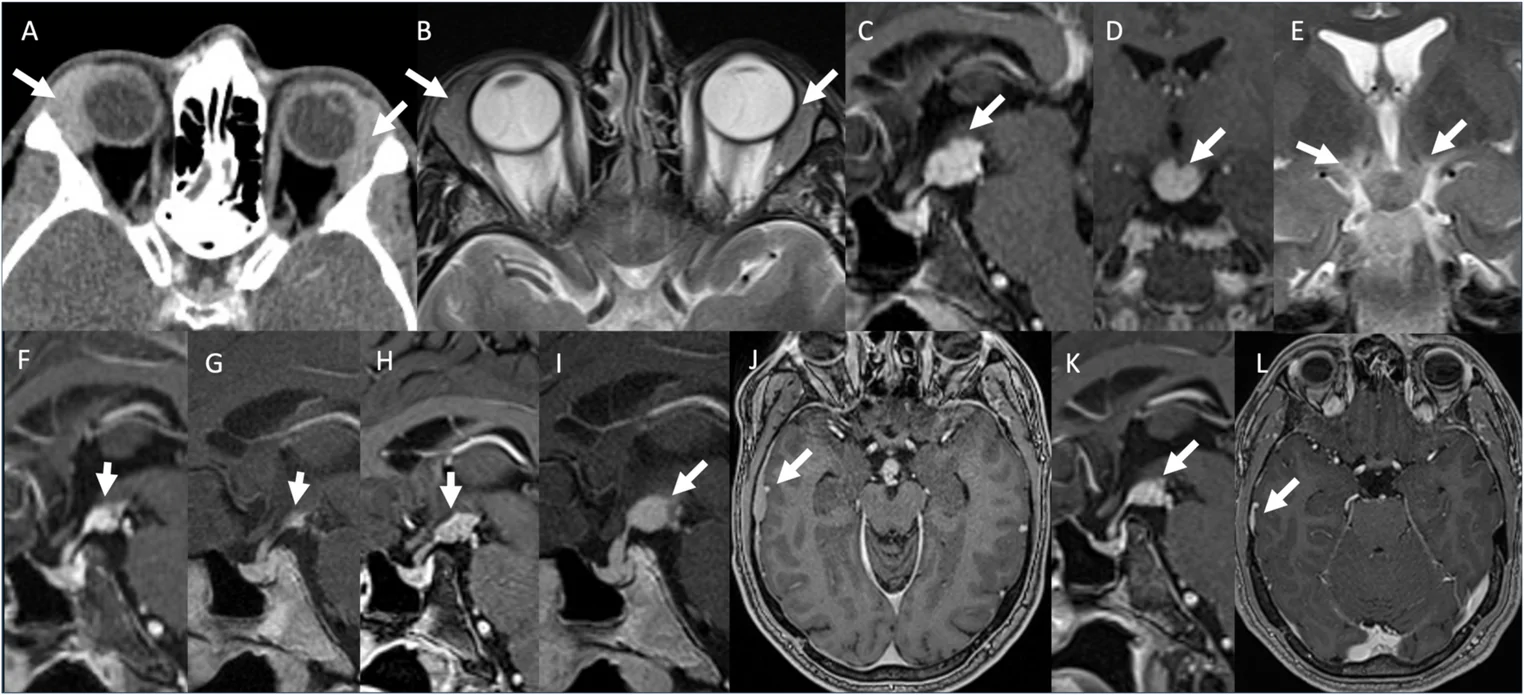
Radiological evolution of hypothalamic and dural involvement in a patient with IgG4-RD. A 33-year-old man with IgG4-RD initially presented with bilateral lacrimal gland enlargement, diagnosed as MALT lymphoma (A, orbital CT; B, orbital MRI; arrows). He subsequently developed hypothalamic involvement associated with hypogonadism (C, sagittal post-contrast T1-weighted image; D, coronal post-contrast T1-weighted image; E, coronal T2-weighted image showing a hypothalamic lesion with mild surrounding edema; arrows), which progressively decreased following treatment with rituximab and chlorambucil (F–G, arrows). At 3-year follow-up, the hypothalamic lesion had increased in size (H–I, arrows), with new right temporal dural thickening (J, arrow). An increase in serum IgG4 levels prompted reassessment of the lacrimal gland biopsy, which showed 80–100 IgG4-positive cells/HPF and an IgG4+/IgG+ ratio of 56–86%, fulfilling the 2019 ACR/EULAR classification criteria for IgG4-RD (24 points). Following treatment with an investigational monoclonal antibody, both the hypothalamic (K, arrow) and dural (L, arrow) lesions decreased in size. Abbreviations: CT, computed tomography; IgG4-RD, IgG4-related disease; MALT, mucosa-associated lymphoid tissue; MRI, magnetic resonance imaging; T1WI, T1-weighted image; T2WI, T2-weighted image
